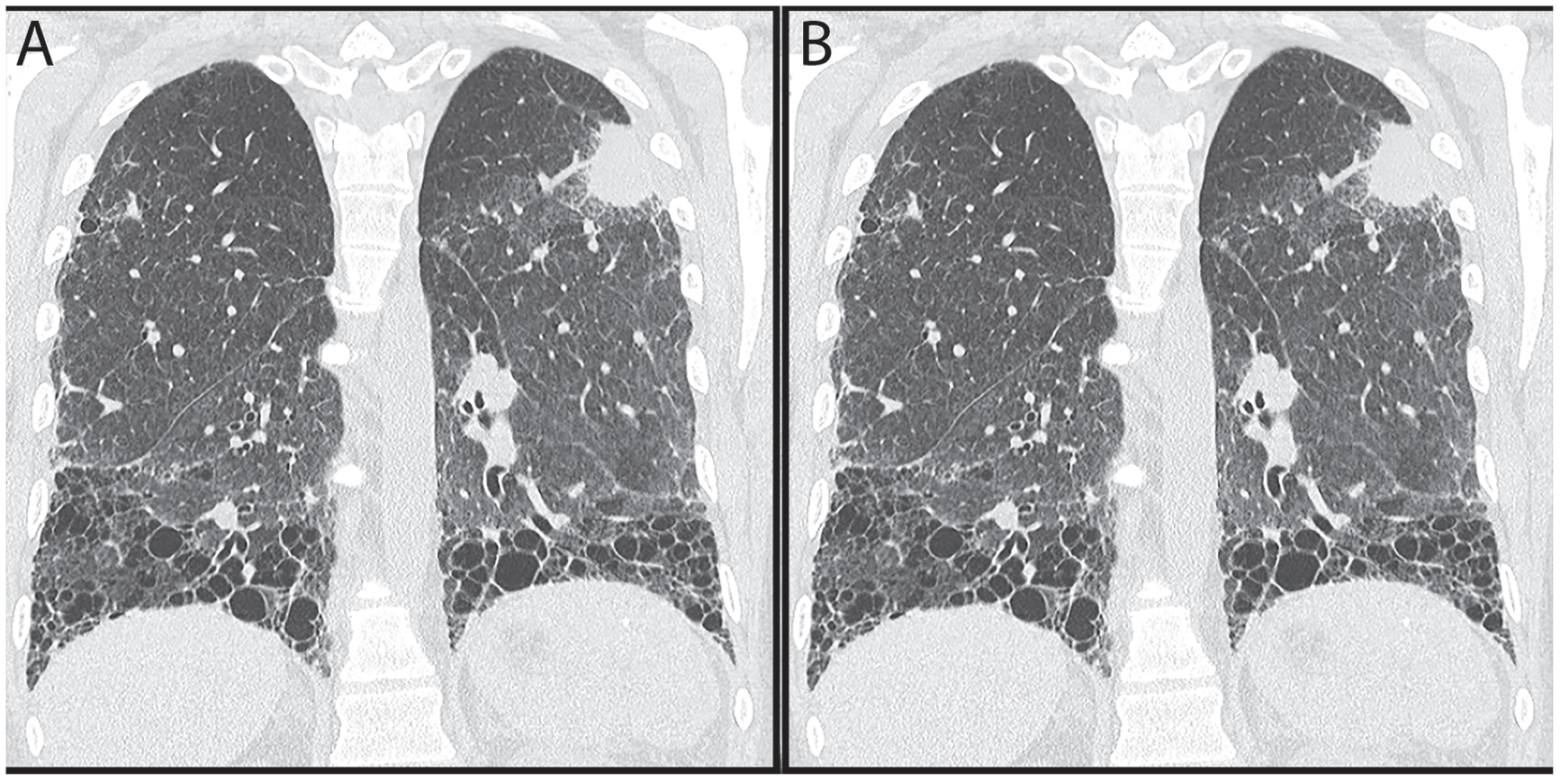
Computed Tomography showing (A) a left upper lobe lung mass with UIP basilar predominant fibrosis and (B) a left lower lobe consolidation (lung cancer) with NSIP.
